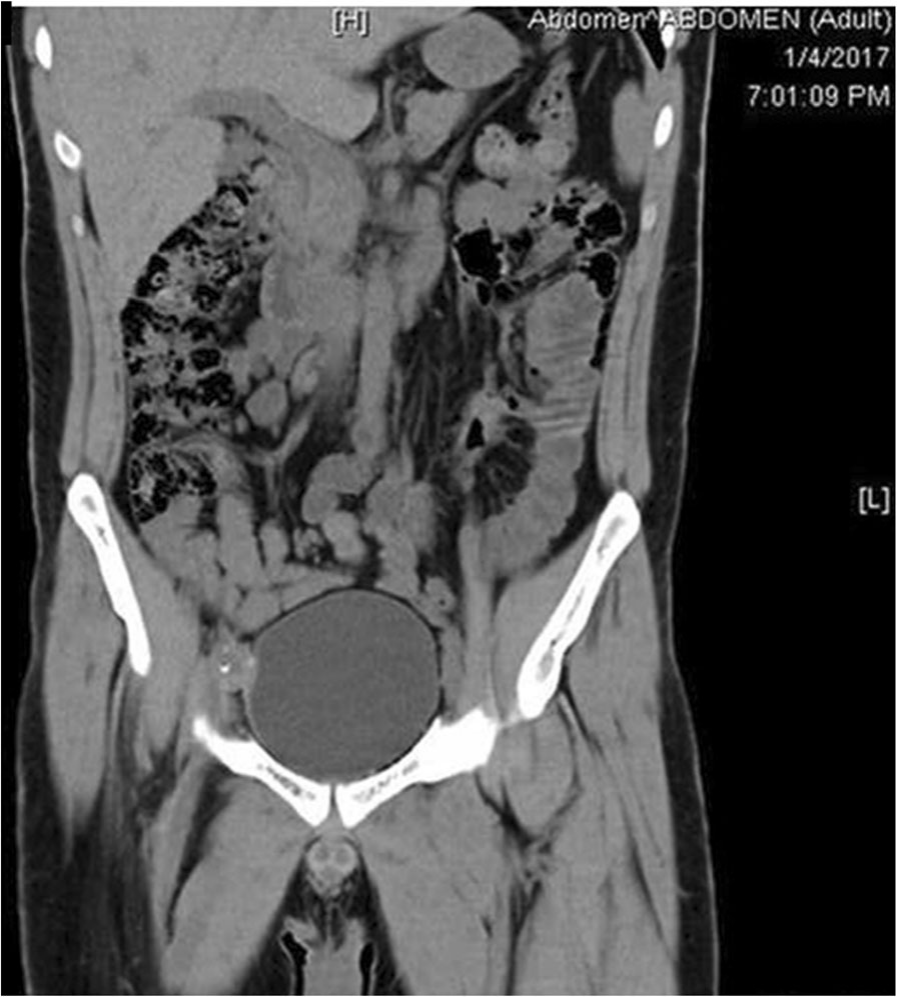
Magnetic resonance imaging showing pseudoaneurysm and the site of rupture at the bladder. Note close proximity of the extra-anatomic graft and urinary bladder
